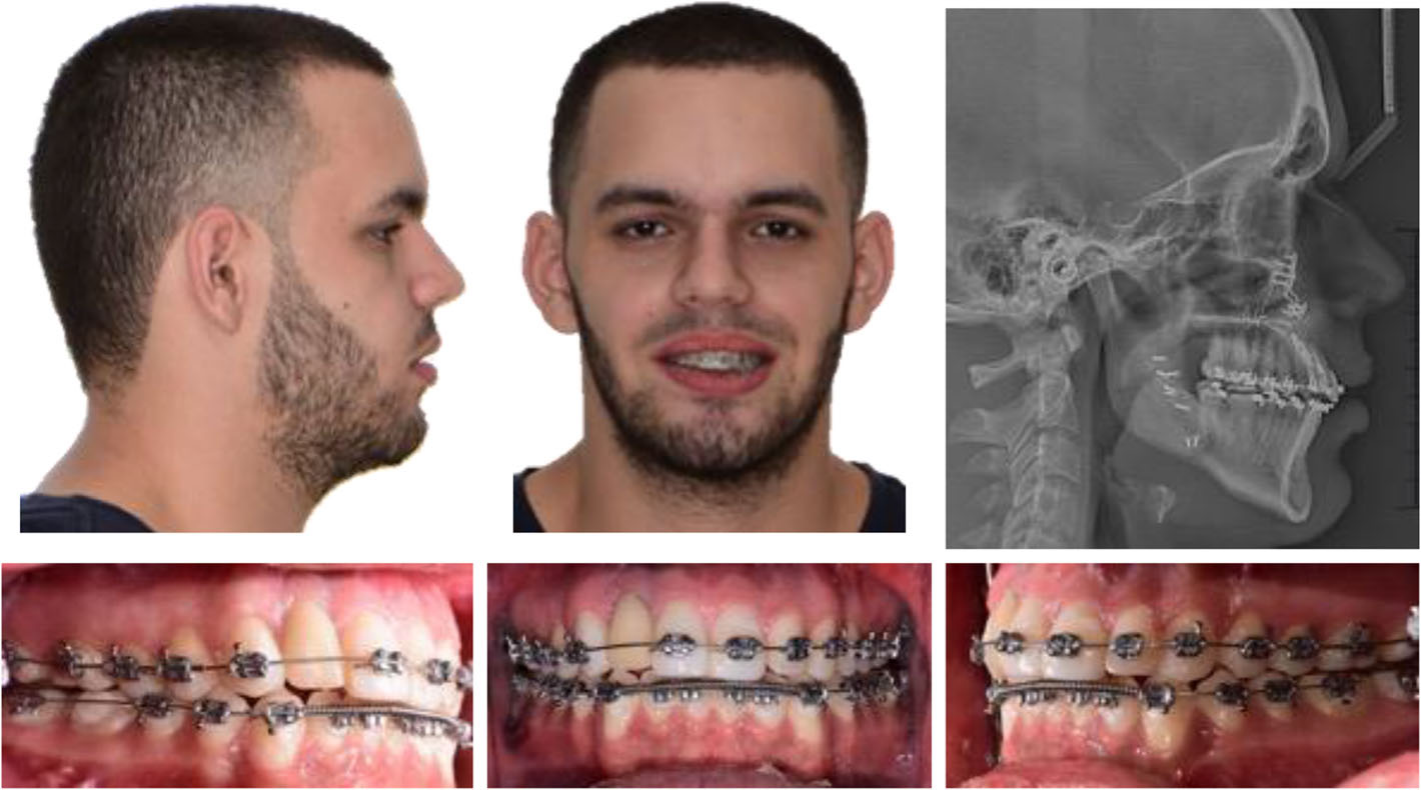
Extra-oral and intra-oral photos, and lateral cephalic X-ray during treatment
